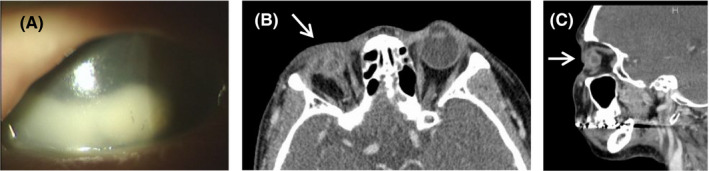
Photograph of the right corneal ulcer at 4 mo after HDR‐ISBT (A). Posttreatment axial (B) and sagittal (C) CT images of the primary tumor. Follow‐up CT scan shows phthisis of the right eyeball (arrows)
